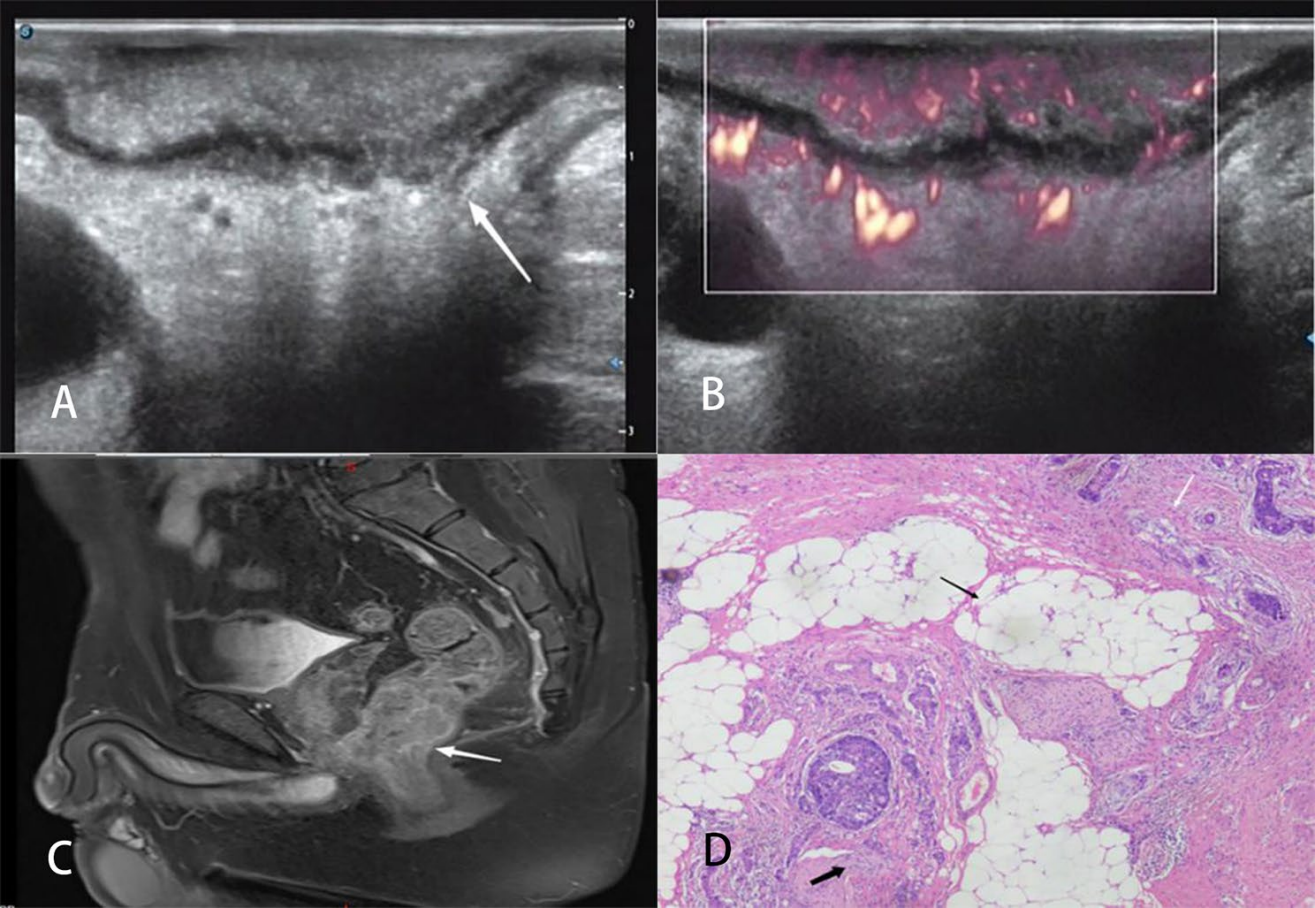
Images of a 42-year-old man with a T4 stage rectal tumor. (A) Two-dimensional endorectal biplane ultrasound of the line array revealed a hypoechoic mass on the rectal wall that invaded the adjacent organs and nearby pelvic tissues (arrow). The diagnosis was T4 rectal cancer. (B) MFI mode image showing that the microblood flow signals of the mass are relatively abundant, with 3–4 short streams distributed below 50% of the maximum section of the tumor. (C) Magnetic resonance imaging revealed thickening of the wall of the lower rectum under peritoneal reflection (arrow); the diagnosis was T4 rectal cancer. (D) The pathologic diagnosis was moderately differentiated adenocarcinoma stage T4 as ulcerative type; the cancerous tissue invaded the adjacent organs and pelvic tissues (white long arrow, serosa; black long arrow, perirectal fat; black short arrow, nerve)
